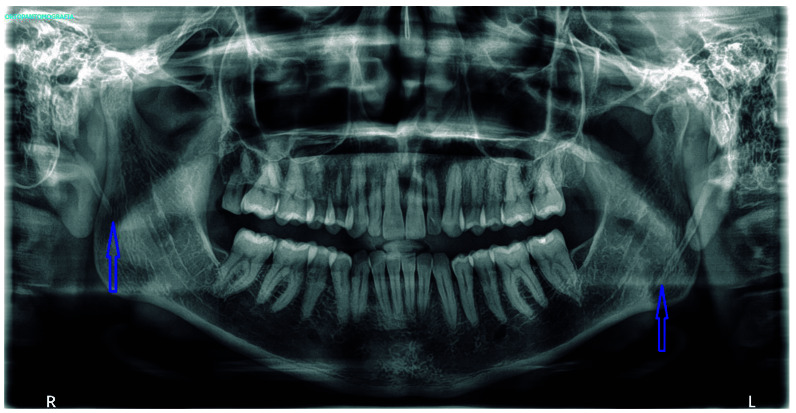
Orthopantomography. Elongated left styloid process (red arrow) compared to the right process (blue arrow).
